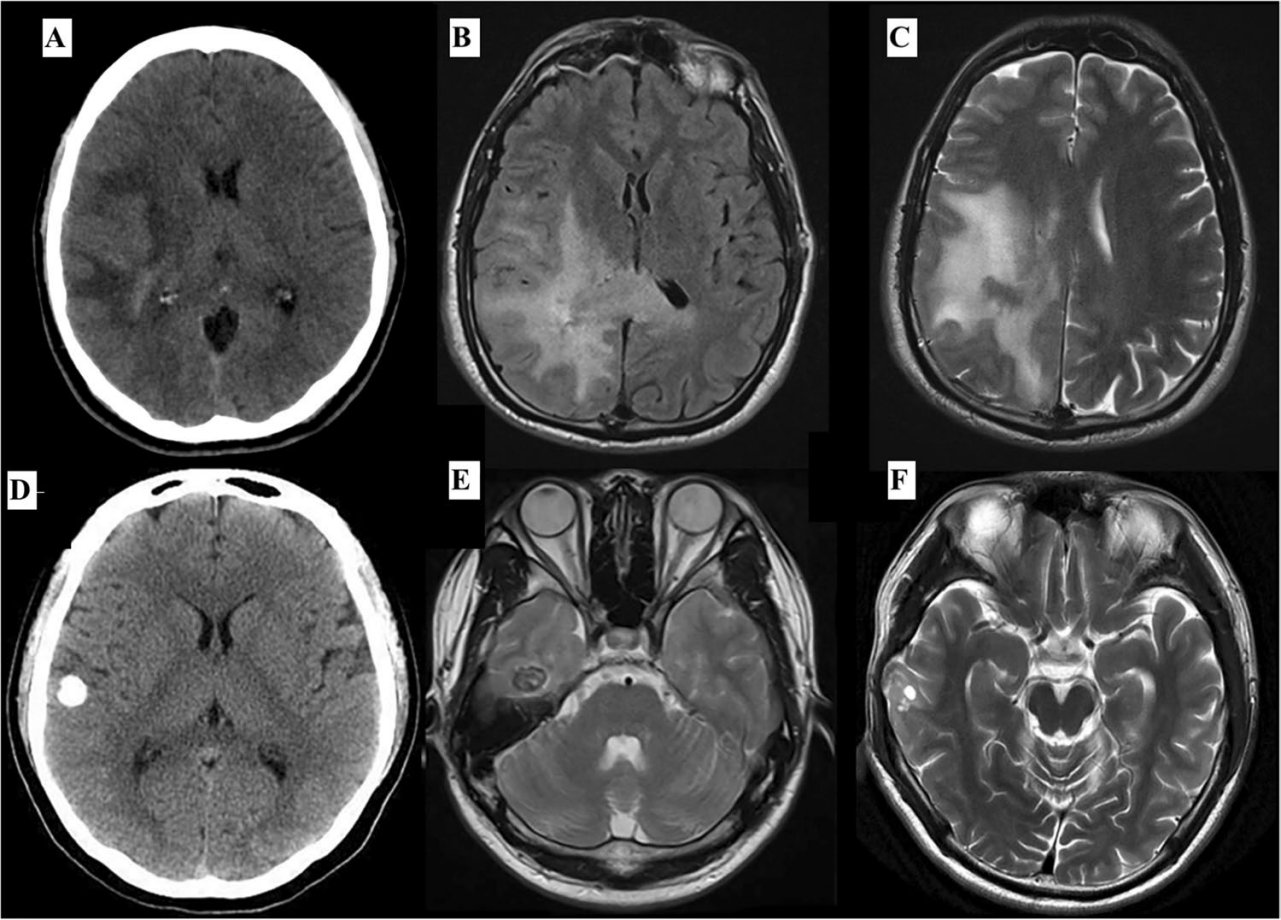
The figure shows the comparison between the radiological images of two patients with a confirmed diagnosis of PLTNY: it is show in particular the high radiological variability that can occur in CT scanning and MRI. In the first patient, a lesion without calcifications (A), with very blurred margins (B) and without cysts in context (C) is documented, while in the second case, a well-localized lesion in the temporal lobe (D) with cysts in context (E) with well-defined margins (F) is appreciated
